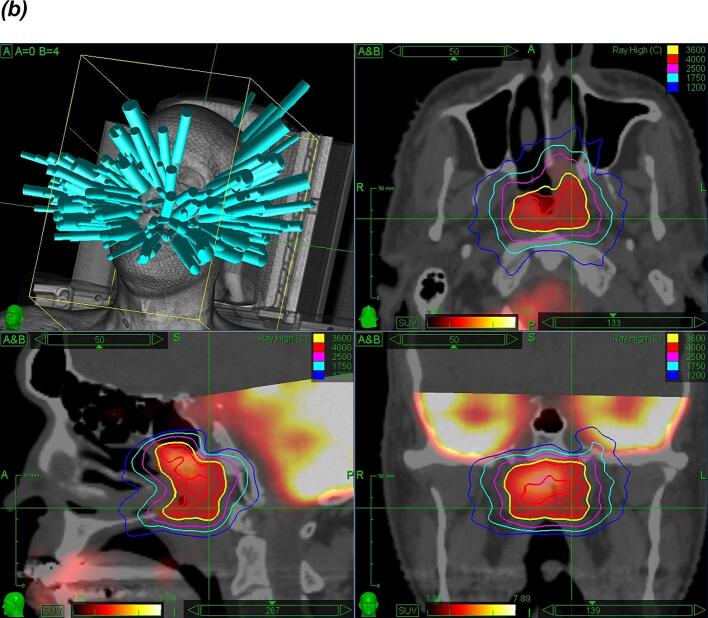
Examples of recurrent tumors treated with stereotactic re-irradiation with a prescription of 6x 6 Gy prescribed on the 85 %-isodose. (a) Excerpt from a linac-based plan of stereotactic radiotherapy with two arcs for a recurrent lymph node after registration with a planning MRI and contouring of the carotid artery (b) Excerpt from a plan of robotic radiosurgery at the Cyberknife-platform with 209 beams from 75 nodes for a recurrent nasopharyngeal cancer after registration with an FDG-PET-CT scan.
